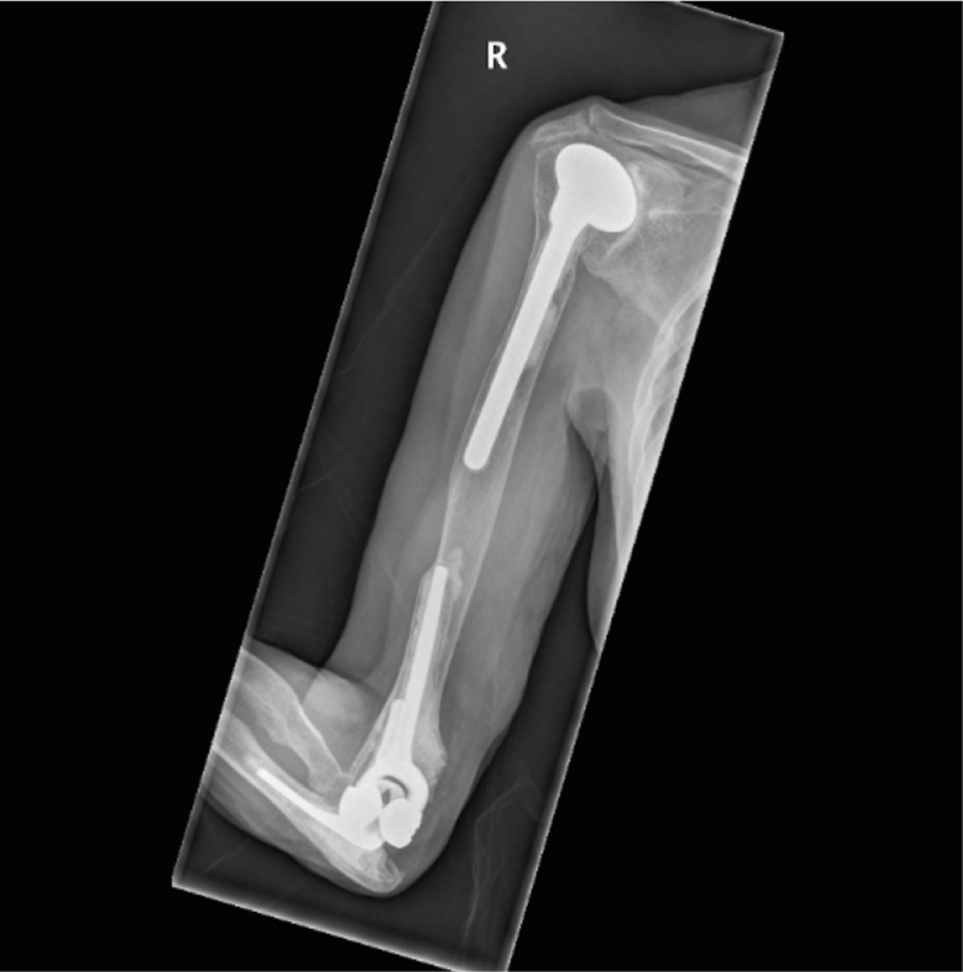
This figure shows the pre-operative radiograph for the third case, demonstrating an elbow replacement and a shoulder hemi-arthroplasty on the same side, with poor bone quality, loosening, and impending fracture.
